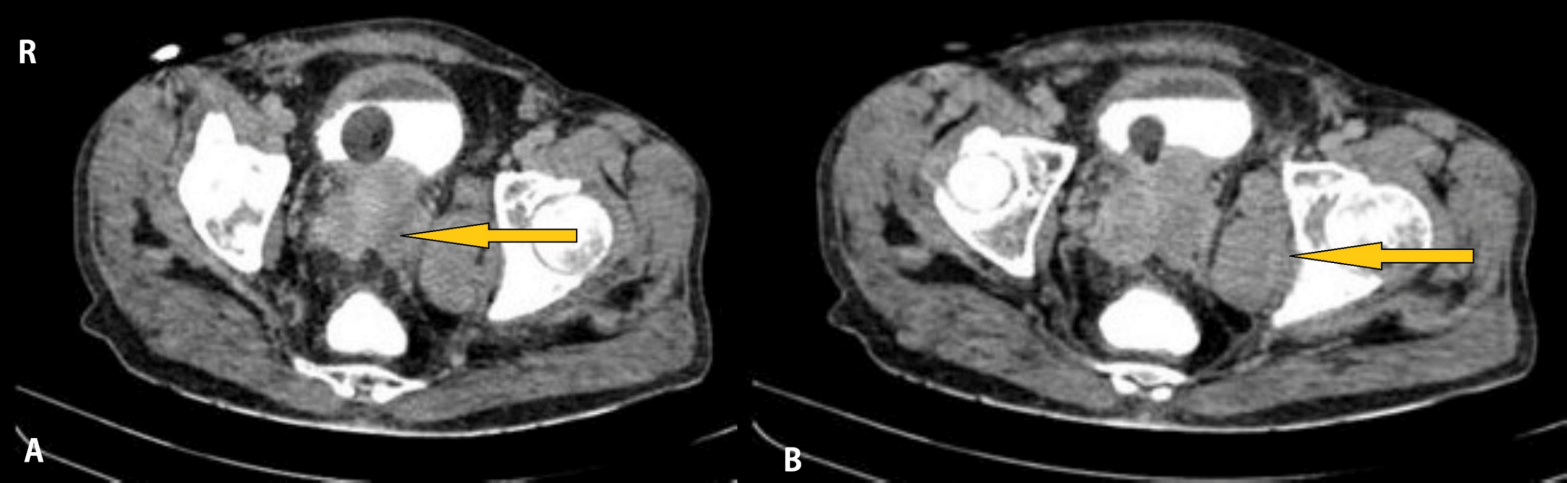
Contrast CT of the abdomen and pelvis showing (A) heterogeneously enlarged prostate with invasion of the surrounding tissue and (B) enhancing deposits in the left internal iliac region and left obturator muscle.
